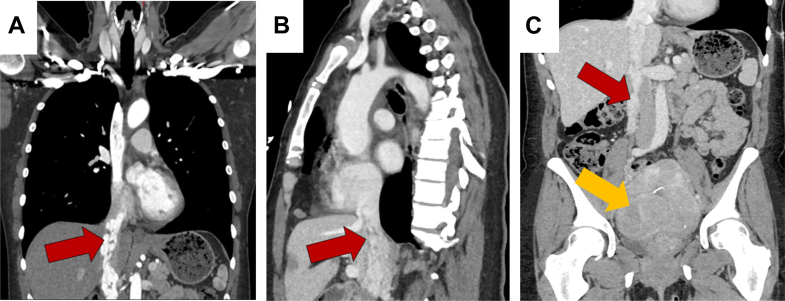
CT Pulmonary Angiogram and CT of the Abdomen/Pelvis Contrast-enhanced CT pulmonary angiogram (A) early phase and (B) late phase, and (C) CT of the abdomen and pelvis demonstrating an extensive intraluminal filling defect extending from the right gonadal vein into the IVC and RA. The red arrow indicates extensive tumor involvement within the IVC, and the yellow arrow denotes the uterine leiomyoma. CT = computed tomography; IVC = inferior vena cava; RA = right atrium.
